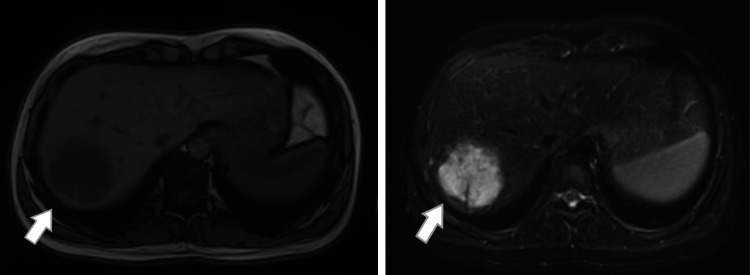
Axial T1 FL2d ΜRI image of the liver and axial T2 fat suppressed MRI image of the liver; white arrow shows a segment VII lesion
